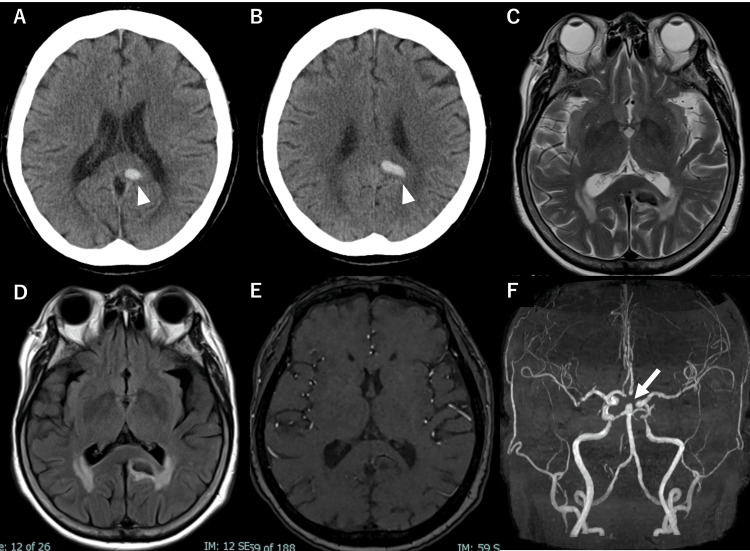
Initial imaging findings Non-contrast computed tomography (A, B) demonstrates intracerebral hemorrhage in the left cingulate isthmus extending to the parietal lobe (white arrowheads). Magnetic resonance imaging shows the hemorrhagic lesion on T2-weighted imaging (C) and fluid-attenuated inversion recovery imaging (D). No clustering of flow voids is observed around the hematoma on T2-weighted imaging, suggesting the absence of an underlying vascular malformation. Time-of-flight magnetic resonance angiography source image (E) demonstrates no abnormal vascular clustering surrounding the hemorrhagic lesion. Three-dimensional reconstructed magnetic resonance angiography (F) demonstrates an incidental basilar tip aneurysm (white arrow).
